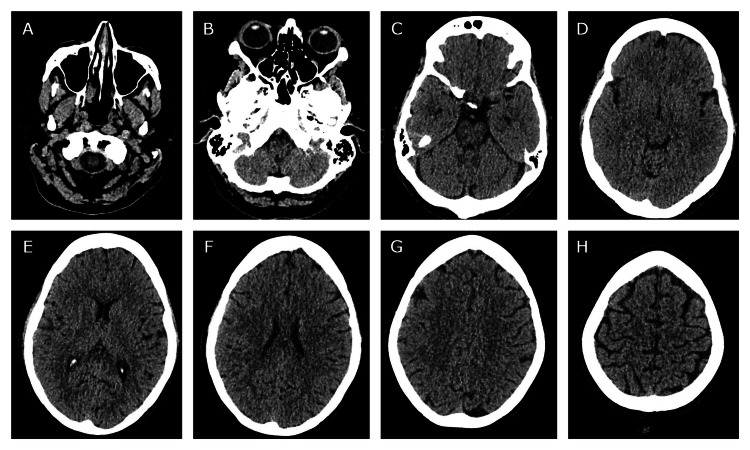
Multiple axial views of a non-contrast CT of the head from the skull base to the vertex in sequential images with no abnormality seen, particularly in the brainstem (A-D).
